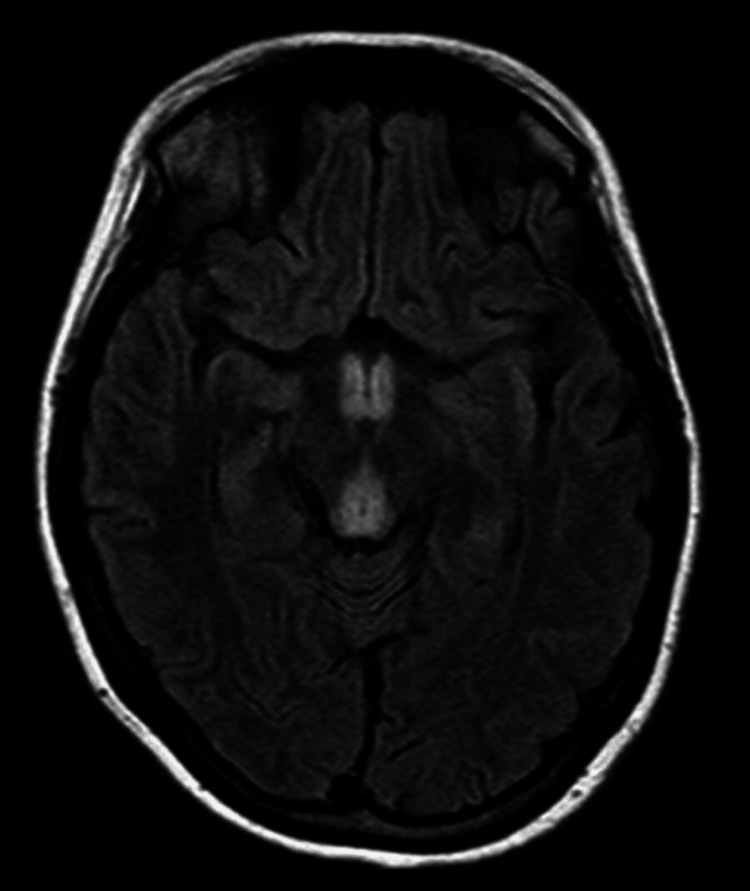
FLAIR MRI showed areas of symmetrical increased signal involving the mammillary bodies, dorsomedial thalami, tectal plate, periaqueductal area and/or around the third ventricle T1 weighted (with or without gadolinium) and DWI images showed no alterations. FLAIR: fluid-attenuated inversion recovery; DWI: diffusion weighted imaging
